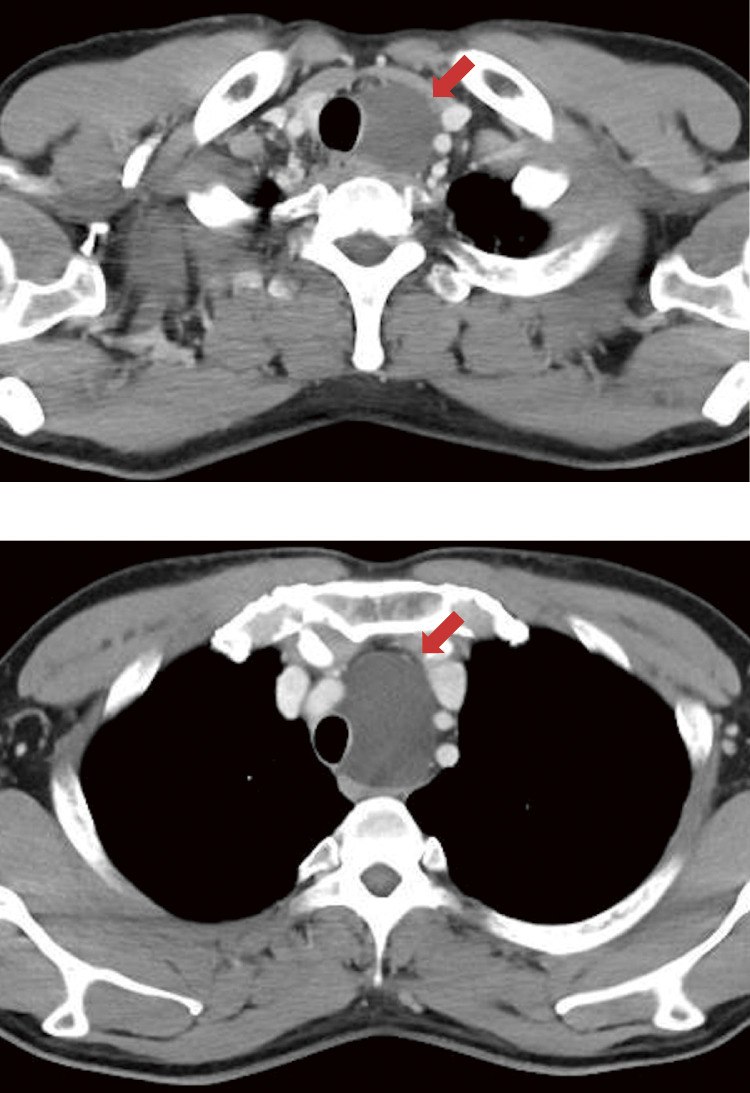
Computed tomography findings. Computed tomography reveals a cystic lesion (arrow) with a maximum diameter of 78 mm, extending from the superior to the middle mediastinum, compressing the trachea to the right.
